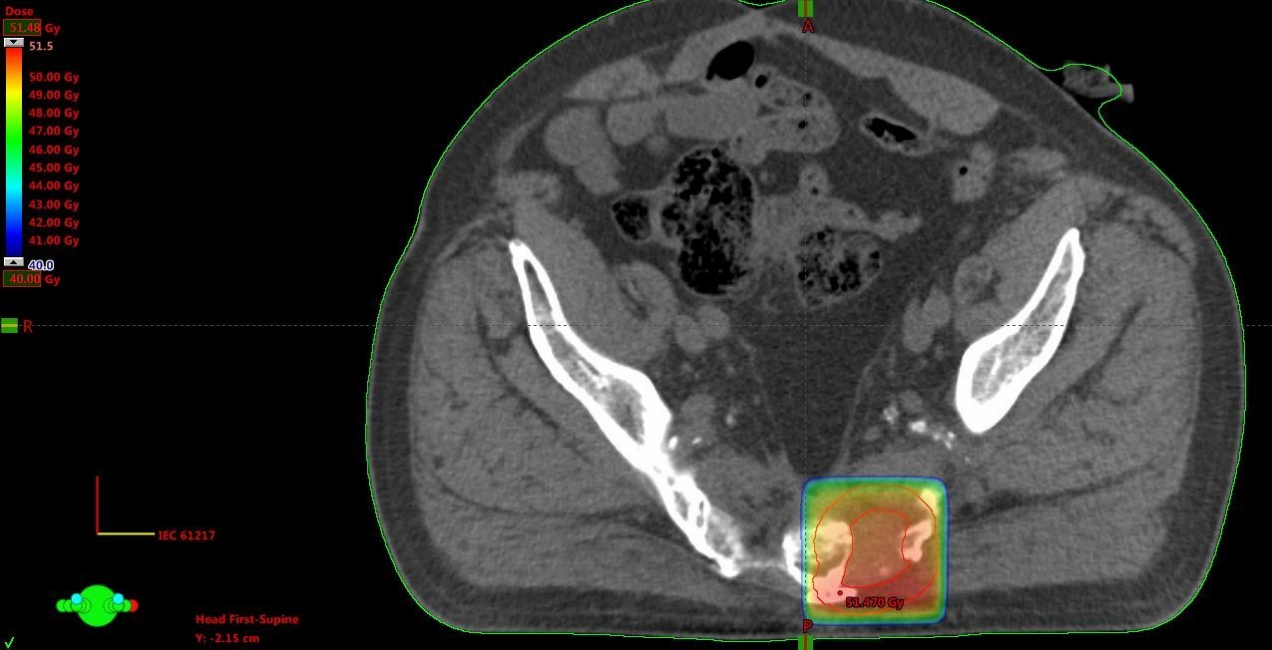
Axial computed tomography (CT) scan and dose distribution from the second course Doses above 40 Gy are displayed, maximum dose 51 Gy.
